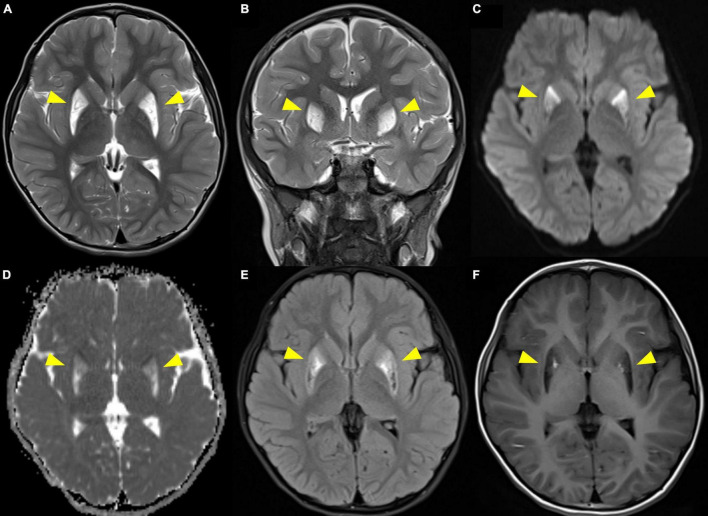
Brain MRI showing high signal intensity on T2-weighted imaging (A. axial, B. coronal) and diffusion-weighted imaging (C), apparent diffusion coefficient (D), and fluid-attenuated inversion recovery (E) images with low signal intensity on T1-weighted imaging (F) in the bilateral putamen. Arrowheads indicate the lesions.
